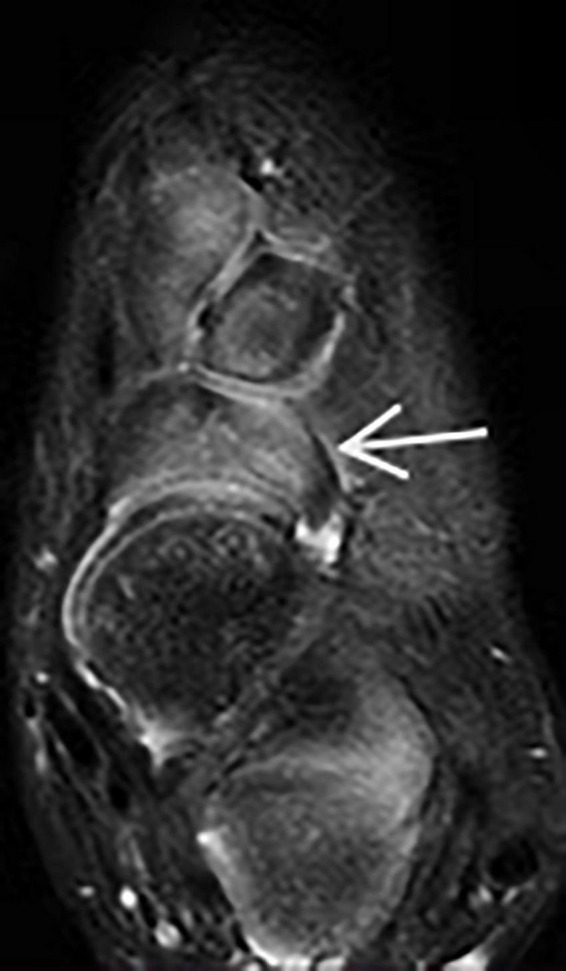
Lateral talonavicular subluxation in the transverse plane of MRI (arrow).
